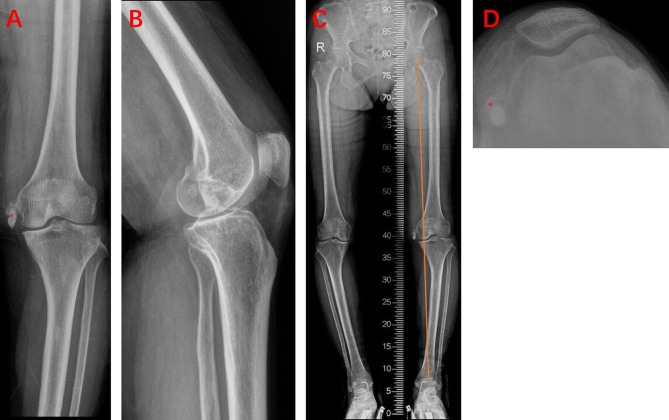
Preoperative x-ray of the left knee. (A) AP view; (B) lateral view; (C) full-weight bearing view; (D) merchant view. *Pellegrini–Stieda lesion.
